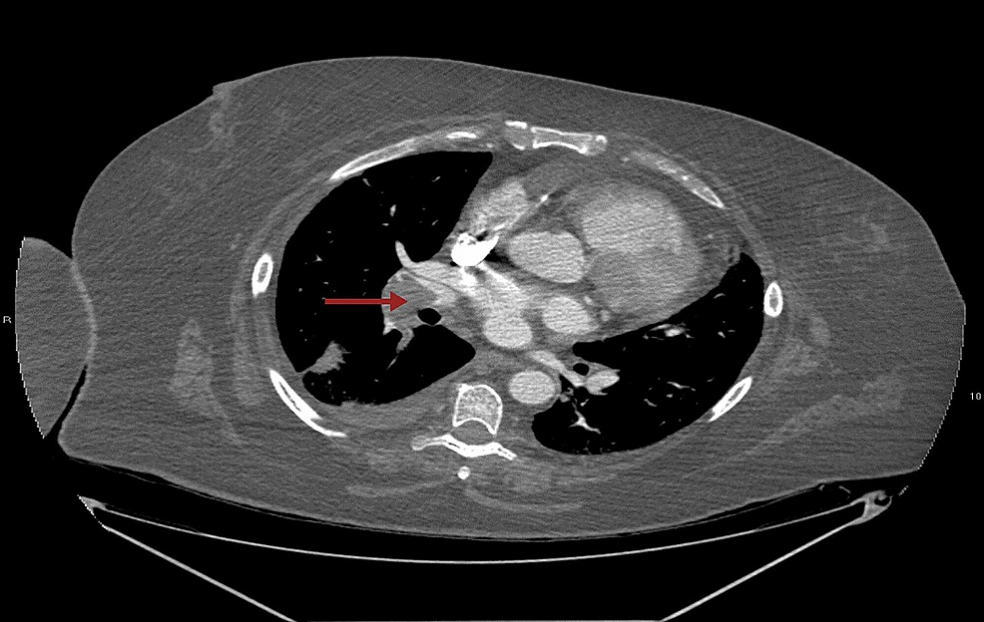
CT angiogram of the chest with the arrow pointing at a large pulmonary embolus within the right interlobar pulmonary artery.
